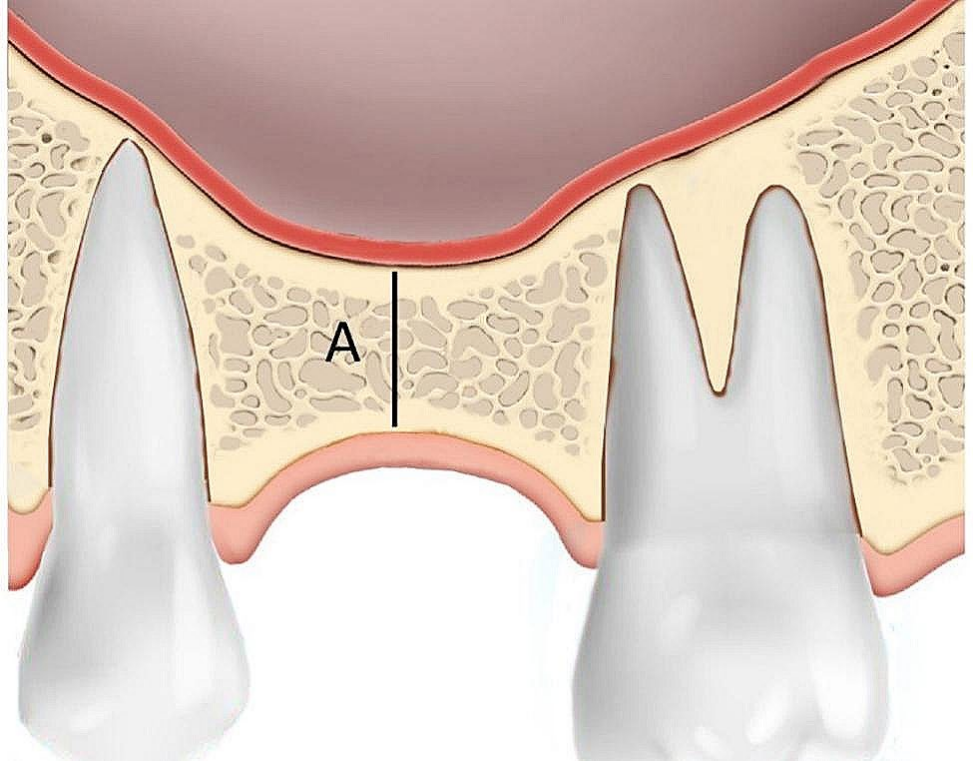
Residual bone height measurement (A); the shortest distance from the alveolar crest to the initial maxillary sinus floor on preoperative CBCT
